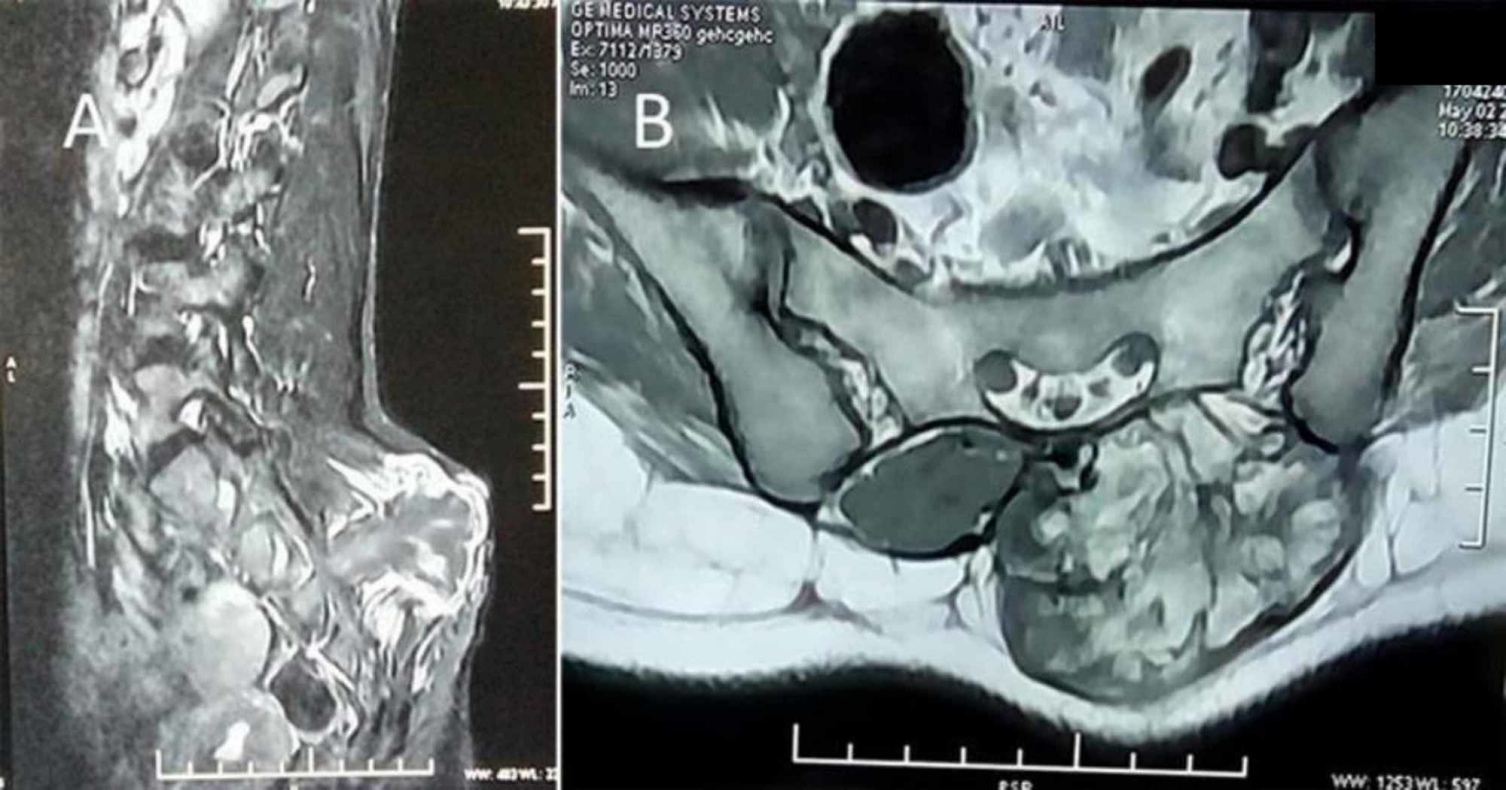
MRI shows a growth with a hypointense rim and a hyperintense center on T1- and T2-weighted images, suggestive of osteochondroma
